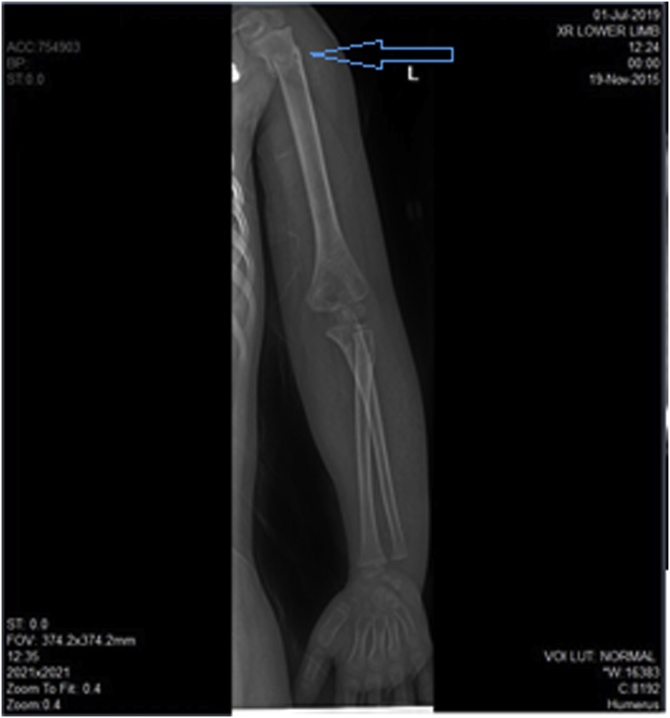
Case 1, plain x-ray left upper limb demonstrated fracture left humerus proximal shaft (blue arrow).
